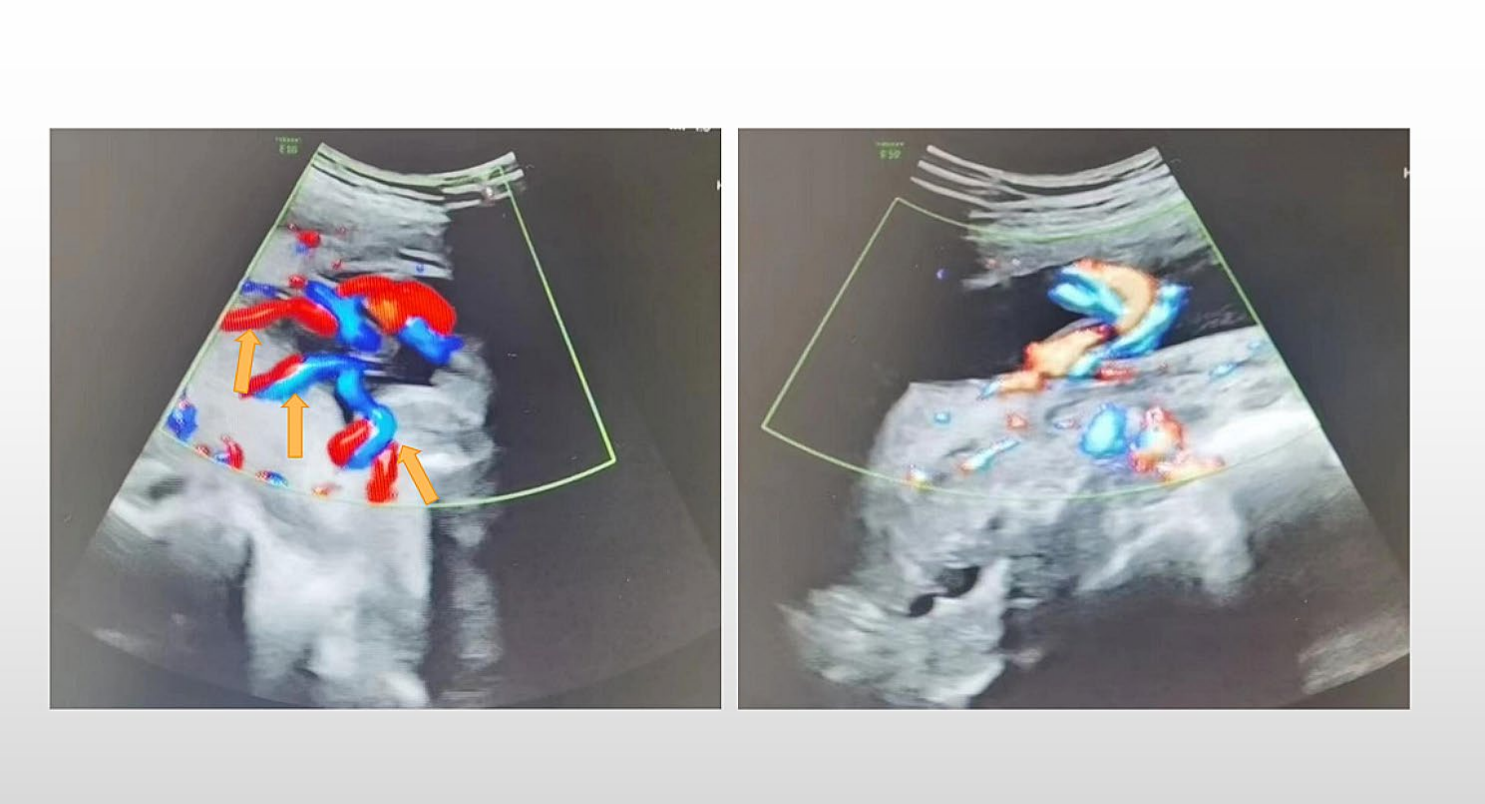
Ultrasonography Images. The vessels indicated by the yellow arrows may have been separated before entering the placenta
